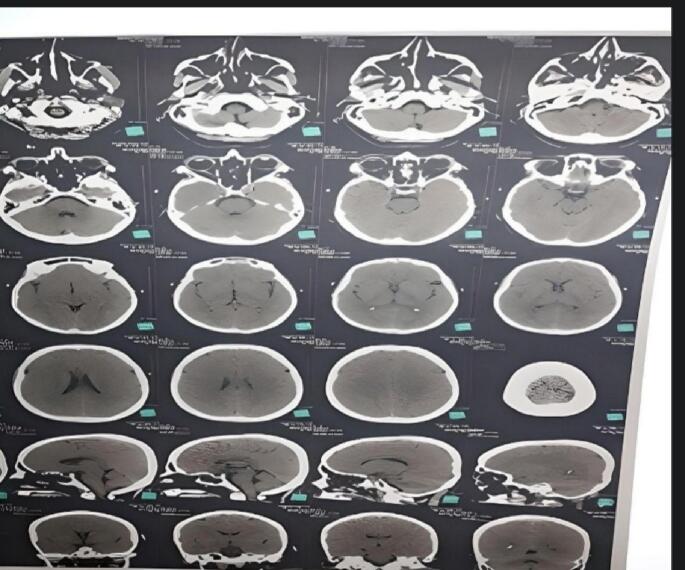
Brain CT scan shows normal tissue.
